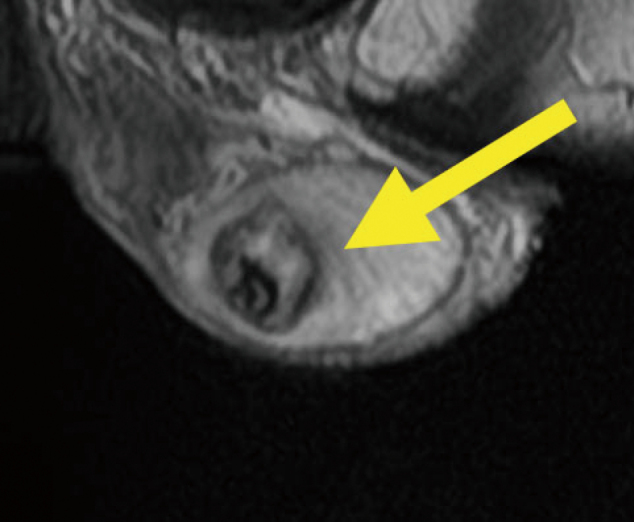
MRI reveals 2.7cm in diameter, clear boundary, and marginally smooth tumor in the right testis
